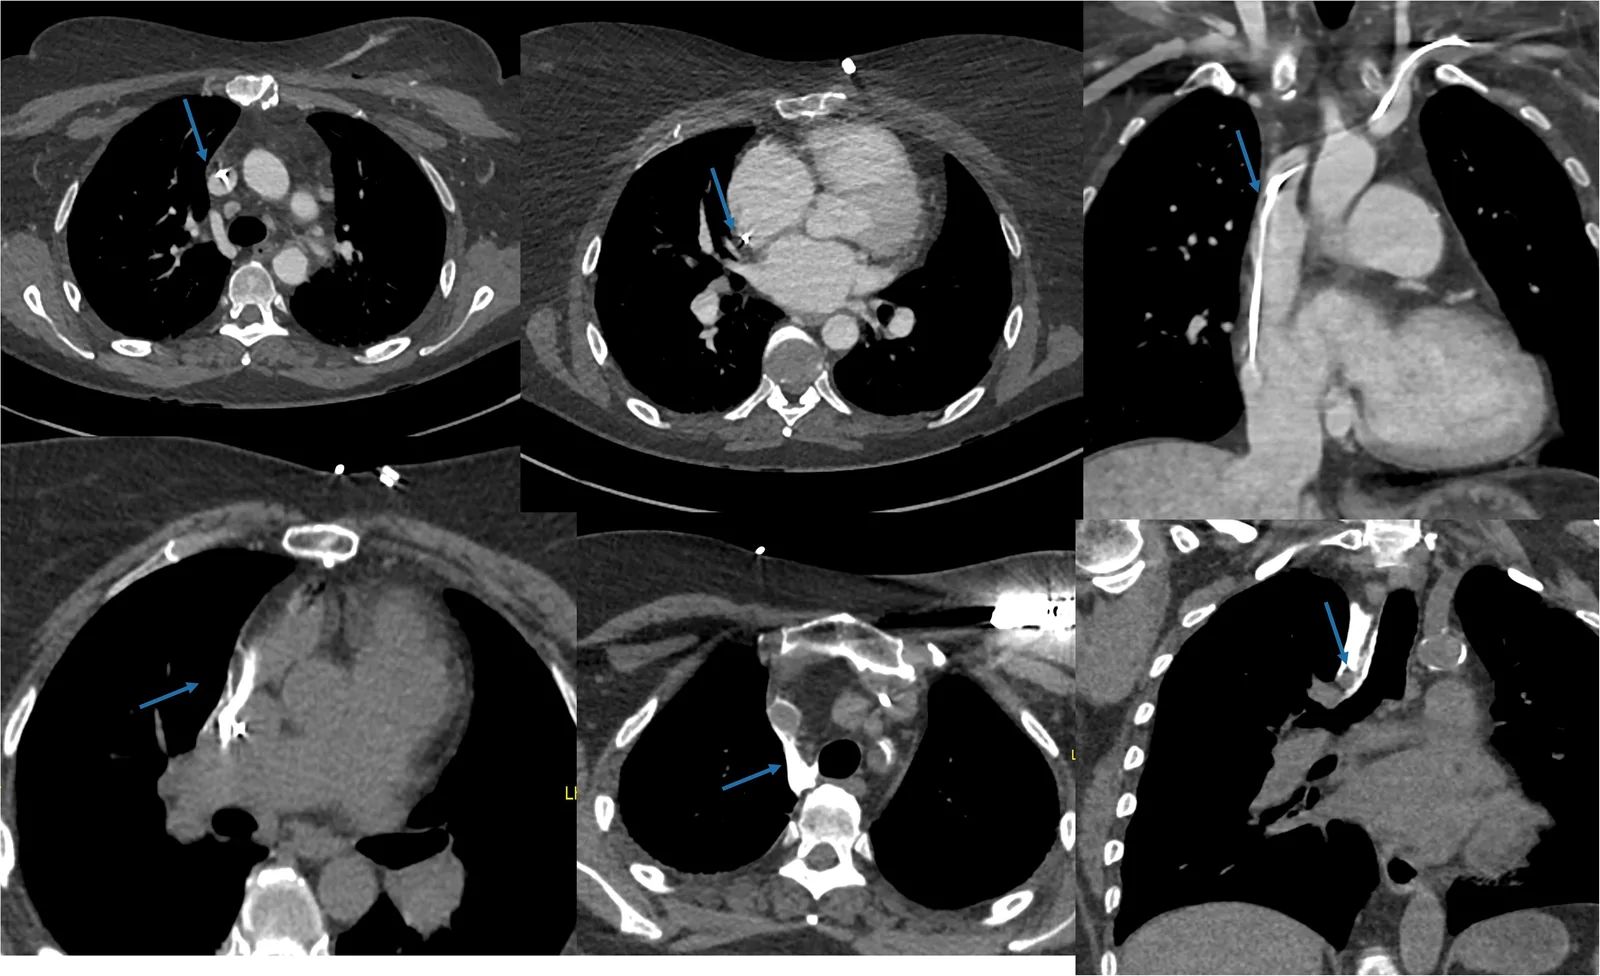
Computed tomography of superior vena cava injury. Axial and coronal non-contrast CT images of the chest demonstrating contrast tracking and mediastinal extravasation adjacent to the superior vena cava at the level of the azygos vein junction (blue arrows), consistent with superior vena cava dissection or contained perforation. The superior vena cava lumen remains patent with no evidence of thrombosis or external compression.
